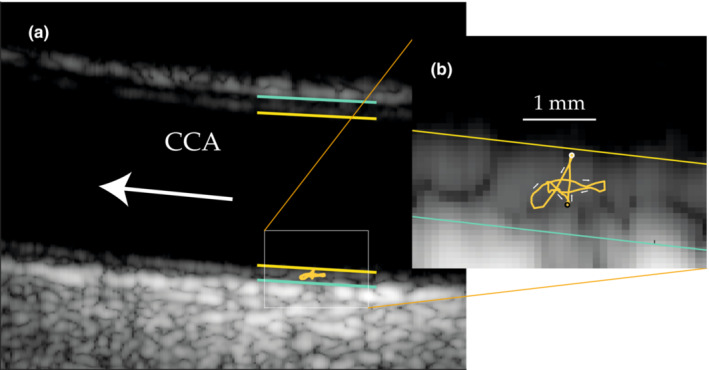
(a) Ultrasound image of the common carotid artery during a measurement of arterial wall motion of a 42‐year‐old female. The yellow lines mark the demarcations between lumen and intima and the green lines the demarcation between media and adventitia. The lines are 5 mm long. The white arrow marks the direction of the blood flow. (b) Enlargement of the far wall. The orange displacement vector curve shows the two‐dimensional wall motion during a cardiac cycle. The longitudinal motion of this vector curve is also shown in Figure 2b. The black circle marks the starting and ending positions as shown in Figure 2b, and the white circle marks the onset of the antegrade motion in early systole. The white arrows mark the direction of the motion. The white line marks 1 mm.
